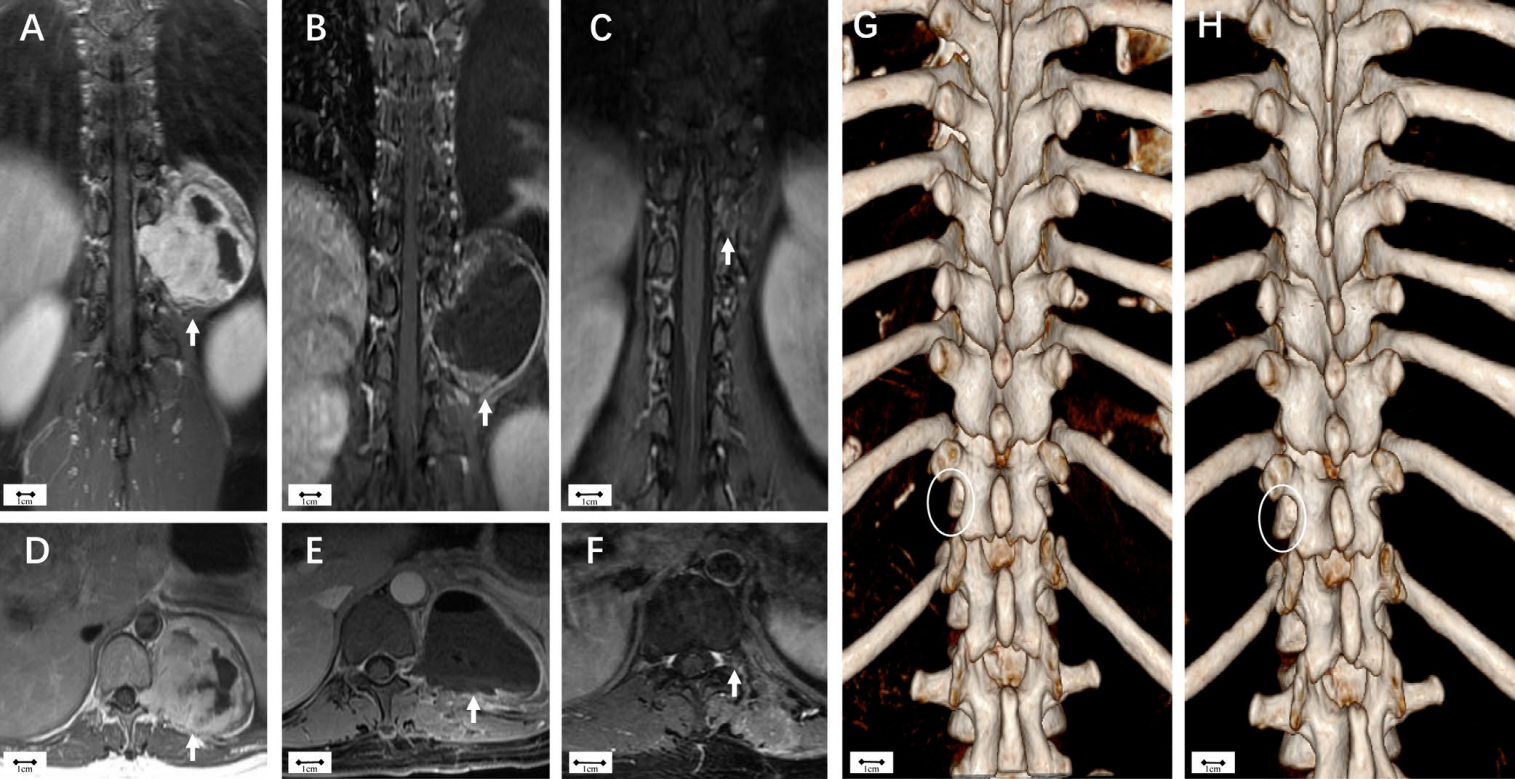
Case I (Eden type IV). (A, D) Preoperative enhanced MRI showed the tumor on the left side of T11/12. (B, E) Postoperative enhanced MRI showed that the tumor was resected entirely through intracapsular. (C, F) The tumor capsule was absorbed, and no recurrence occurred during the 2‐year follow‐up period. (G, H) Postoperative and 2‐year follow‐up CT‐VRT‐3D reconstructions revealing no impact on the spinal bone integrity (circle).
